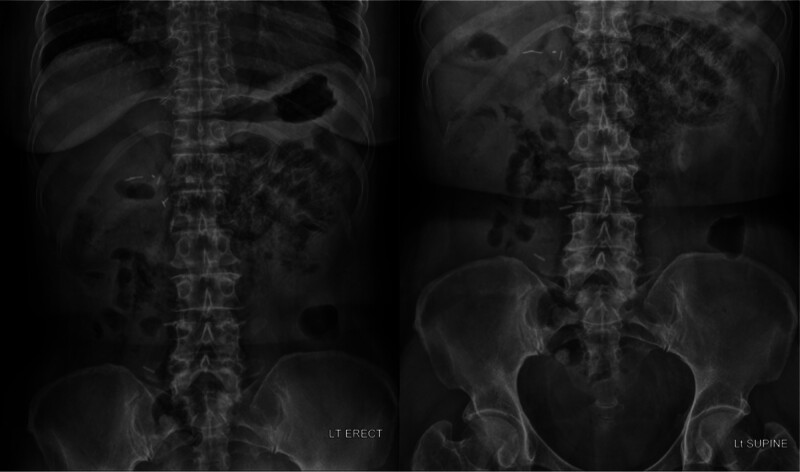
Plain X-ray of the abdomen (erect and supine) performed on the third day of hospitalization.
